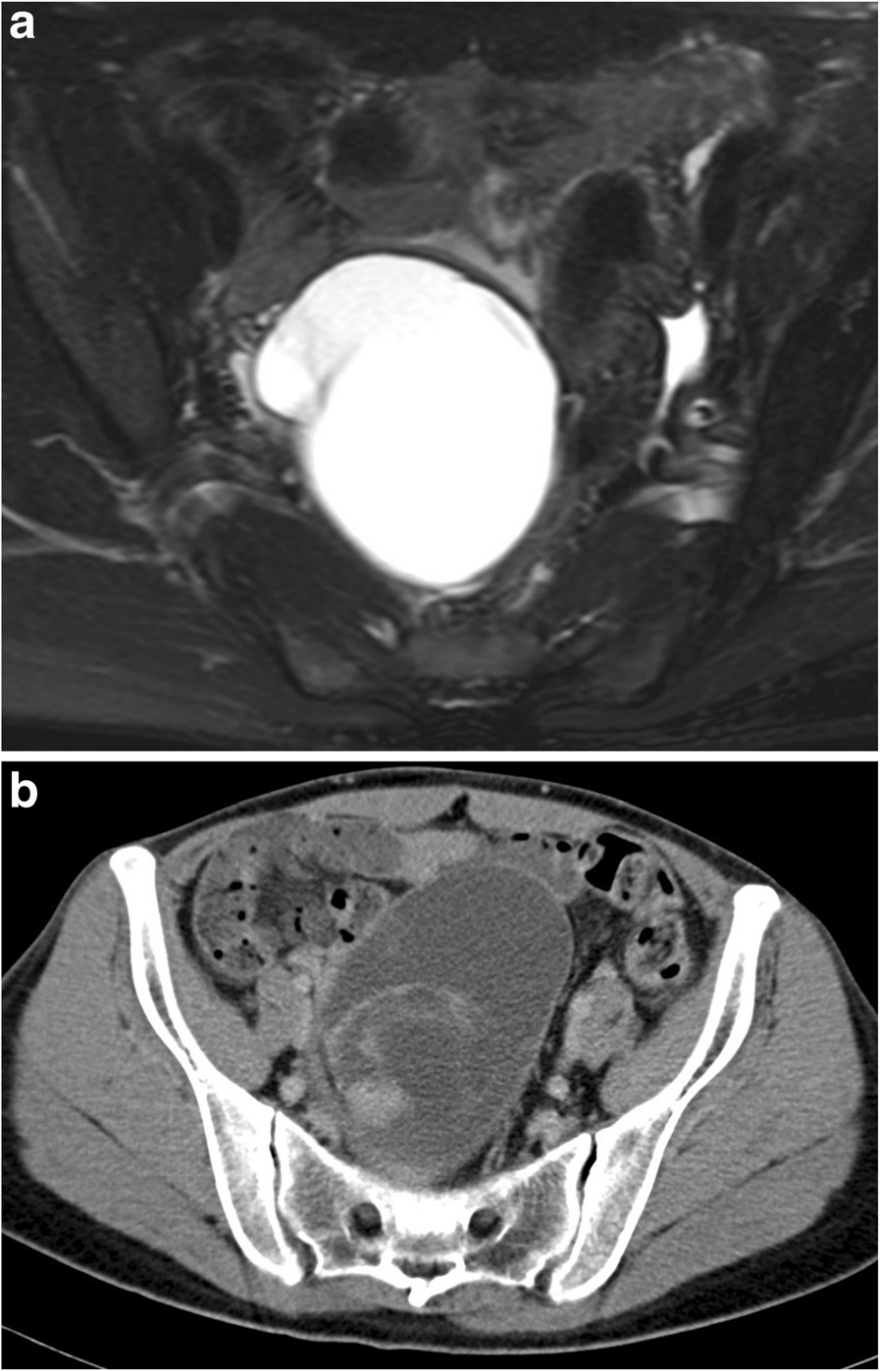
Image analysis. a) Gadrinium-enhanced T1 weighted magnetic resonance imaging shows a multilocular cystic lesion in the right ovary. b) Enhanced computed tomography shows an intraabdominal mass lesion occupying the pelvic cavity. Intracystic irregular enhancement was focally noted.
